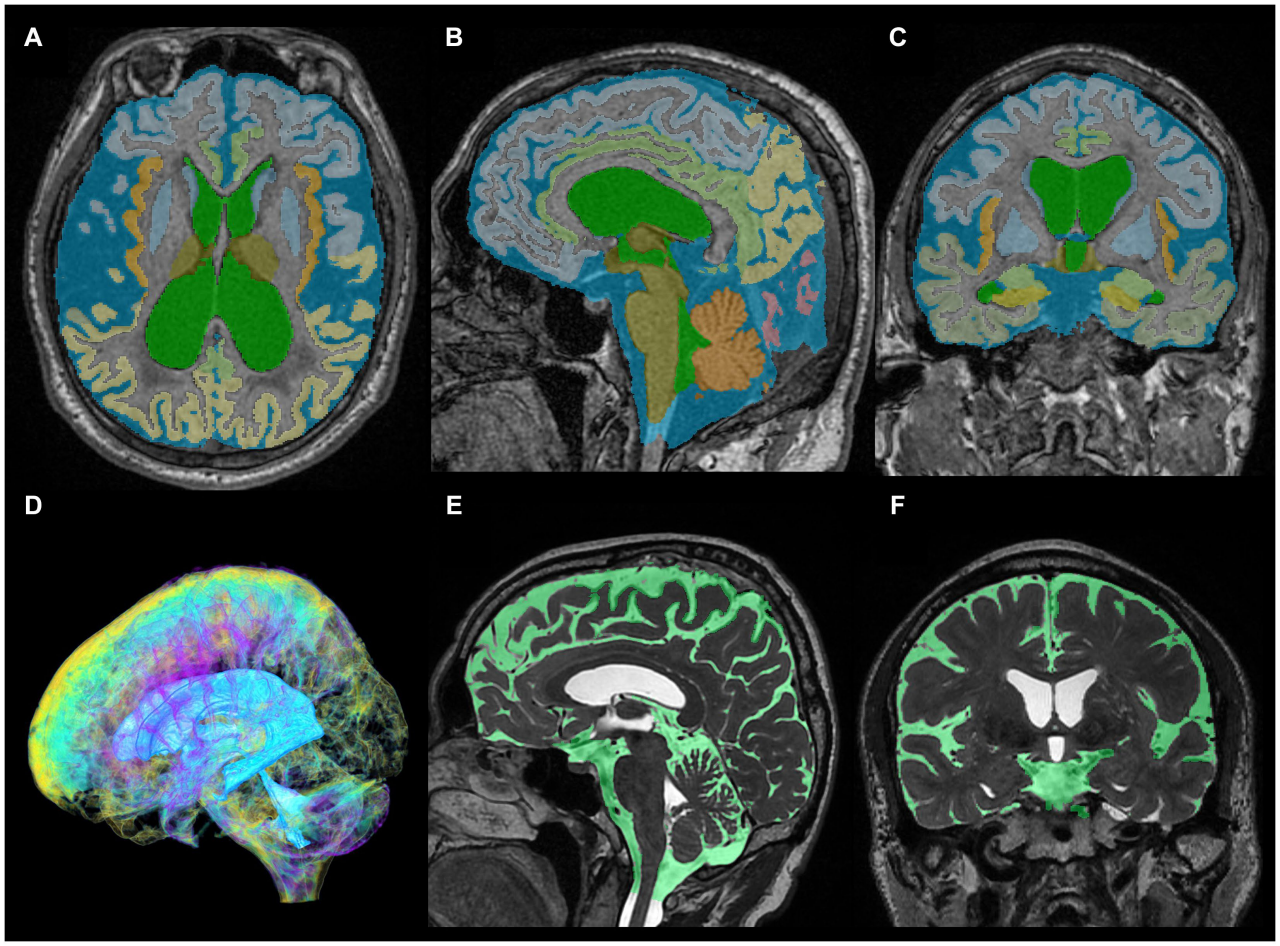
Segmentation from three-dimensional T1- and T2-weighted MRI. The upper axial (A), sagittal (B), and coronal (C) images on 3D T1-weighted MRI show the results for fully automatically segmented regions, including total ventricles (green) and total subarachnoid spaces (marine blue) of a representative patient with Hakim disease and DESH, using the Brain Subregion Analysis application on the 3D volume analyzer SYNAPSE 3D workstation (FUJIFILM Corporation). The lower three-dimensional (D), sagittal (E), and coronal (F) images on 3D T2-weighted MRI show the results of manually segmented total ventricles (light blue in D) and total subarachnoid spaces (light green in E,F) of a representative healthy elderly volunteer.
